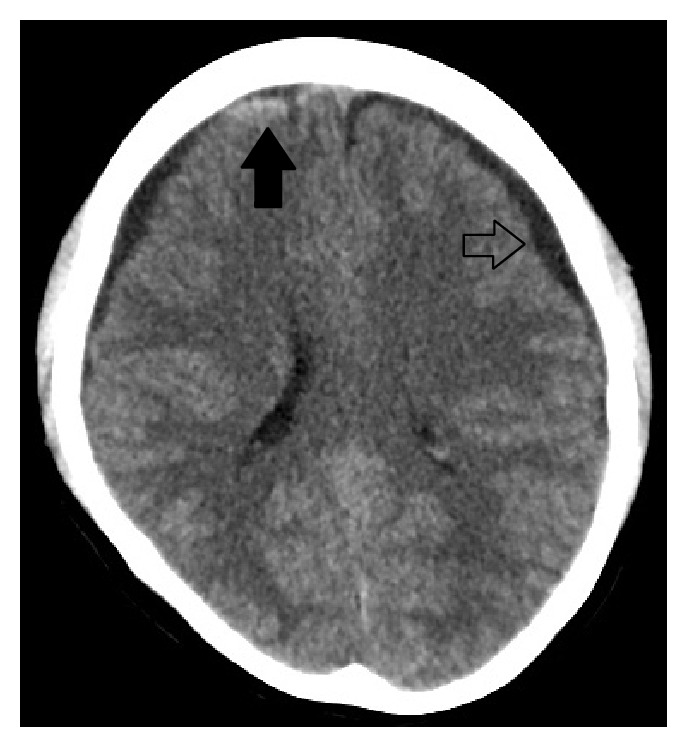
Nonintravenous contrast enhanced brain CT demonstrates bilateral CSF-density subdural hygromas (left subdural hygroma labelled with an open arrow) and a hyperdense acute right frontal subdural hematoma (solid arrow).
